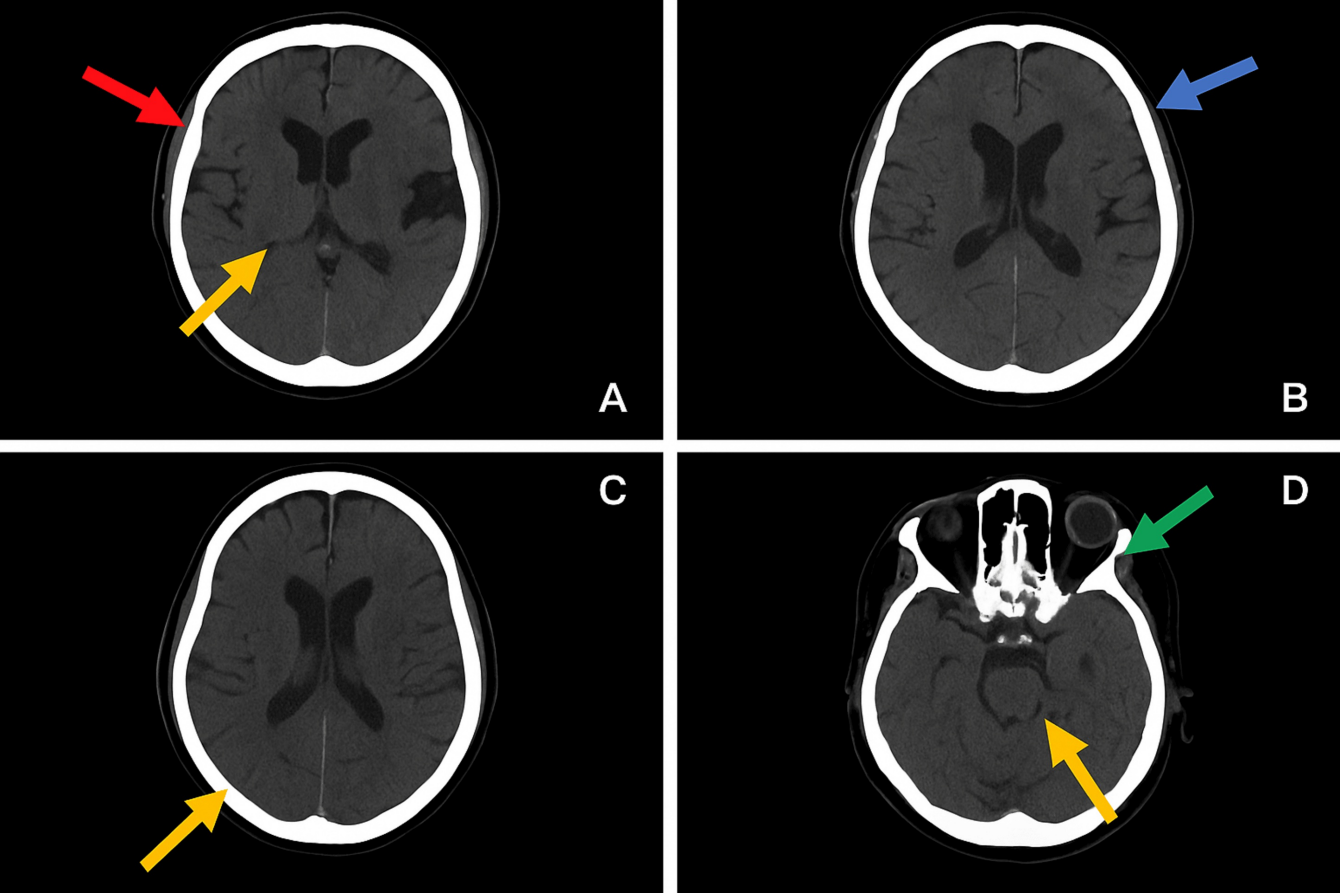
Non-contrast CT of the brain. Axial non-contrast CT images of the brain (A–D) demonstrating no intracranial hemorrhage, no abnormal parenchymal densities, and no midline shift. The ventricular system and subarachnoid spaces appear within normal limits. Mild widening of the subarachnoid space at the vertex is noted. A partially empty sella is present. Several ethmoidal air cells show opacification, while the remaining paranasal sinuses are well aerated. Impacted maxillary teeth projecting into the maxillary sinus floor are visualized. Linear hyperdense subcutaneous structures in the occipital region create streak artifacts. A: Red arrow = widening of the subarachnoid space at the vertex; yellow arrow = partially empty sella. B: Blue arrow = prominent cortical sulci. C: Yellow arrow = prominence of subarachnoid space. D: Green arrow = opacification of ethmoidal air cells; yellow arrow = impacted maxillary tooth projecting into the maxillary sinus floor.
